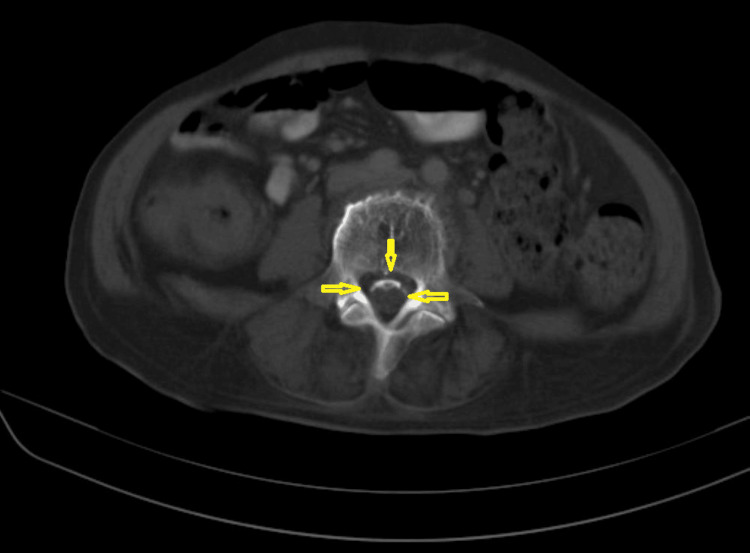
CT is the best investigation tool to visualize the ossified intraspinal lesion and nerve roots. There are curvilinear dural calcifications, intrathecal coarse calcifications, and ossified individual nerve roots adhered to the posterior thecal sac. (Image published under Creative Commons License; image credits: case courtesy of Noriza Zainol Abidin, Radiopaedia.org, rID: 51394)
